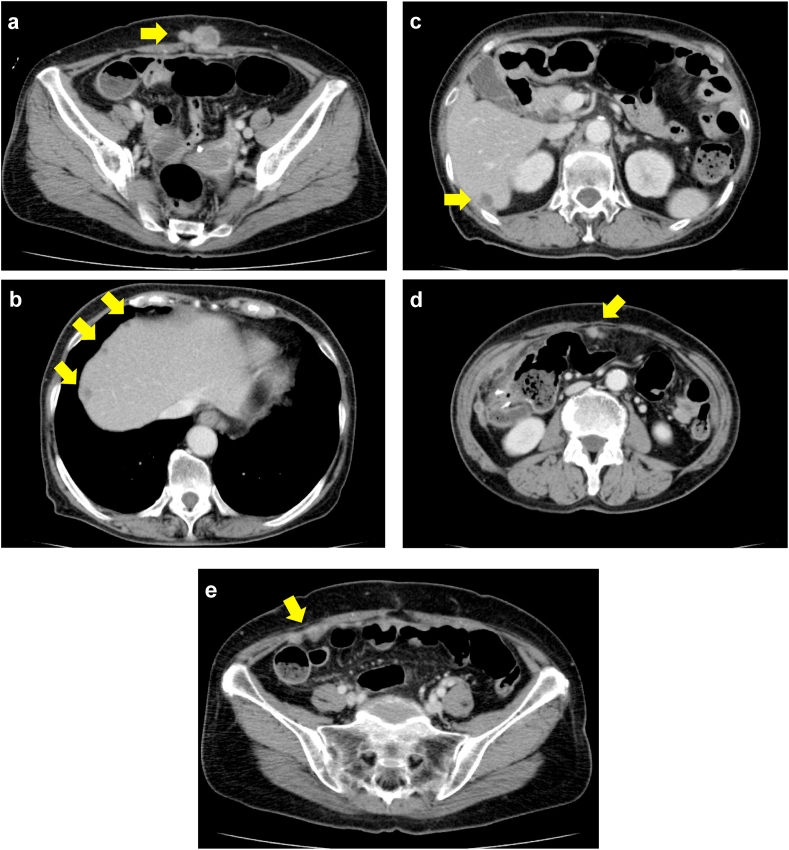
Computed tomography findings of cancer recurrence. a: abdominal wall metastases, b, c: multiple liver metastases, d, e: multiple peritoneal metastases.
